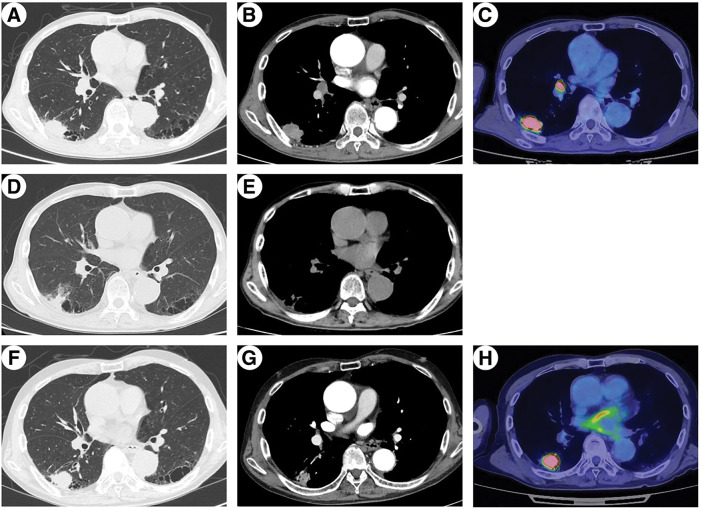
Chest CT and FDG-PET. Images demonstrate tumor response to neoadjuvant chemoimmunotherapy and subsequent regrowth. (A–C) Pre-neoadjuvant chemoimmunotherapy chest CT/FDG-PET showing a 42-mm right lung tumor and FDG uptake in the No.11i lymph node. (D, E) Chest CT before steroid treatment showing tumor shrinkage (19 mm) and reduced No.11i lymph node. (F–H) Preoperative chest CT showing regrowth of tumor (33 mm), whereas No.11i lymph node remained reduced in size, making preoperative biopsy difficult. FDG, fluorodeoxyglucose
